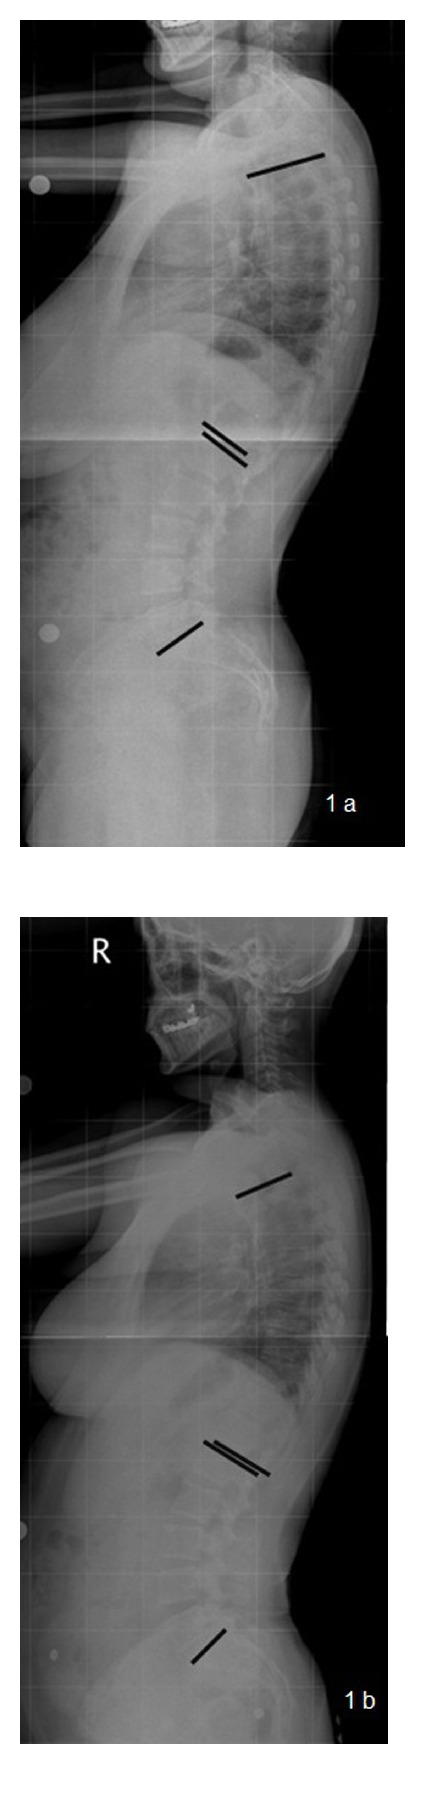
(a) Preoperative lateral X-ray of a 38-year-old patient. The black lines are the landmarks for the measurements of thoracic kyphosis and lumbar lordosis angles. (b) Postoperative (14 months) lateral X-ray of the same patient and used vertebral landmarks for measurements.
